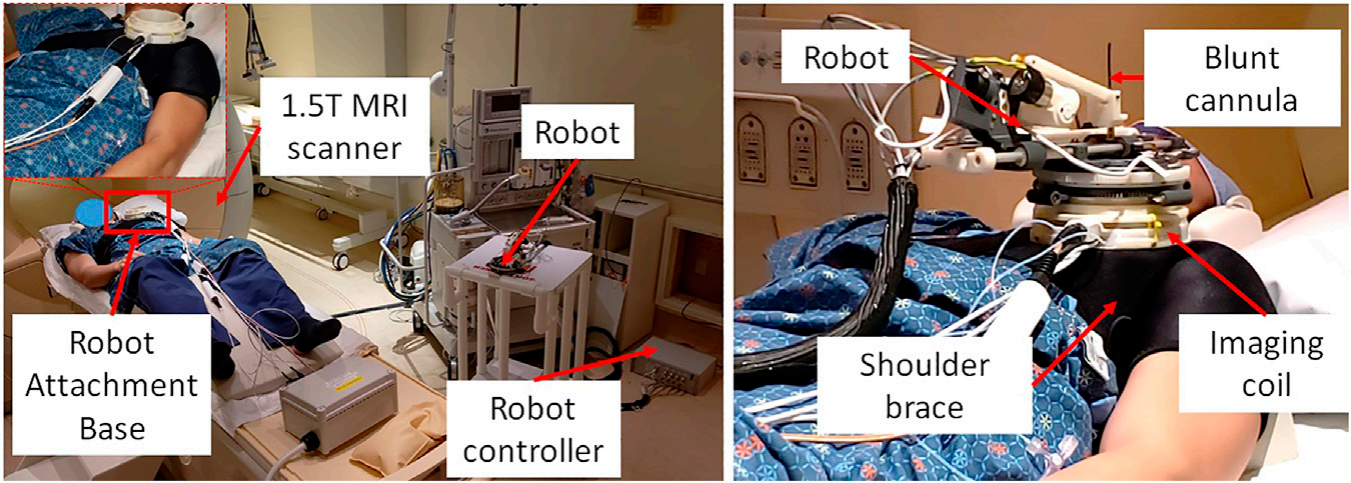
System setup for one of the volunteer study showing (left) placement of system components in an interventional MRI suite and (right) robot attached on the shoulder of the volunteer using a shoulder brace.
